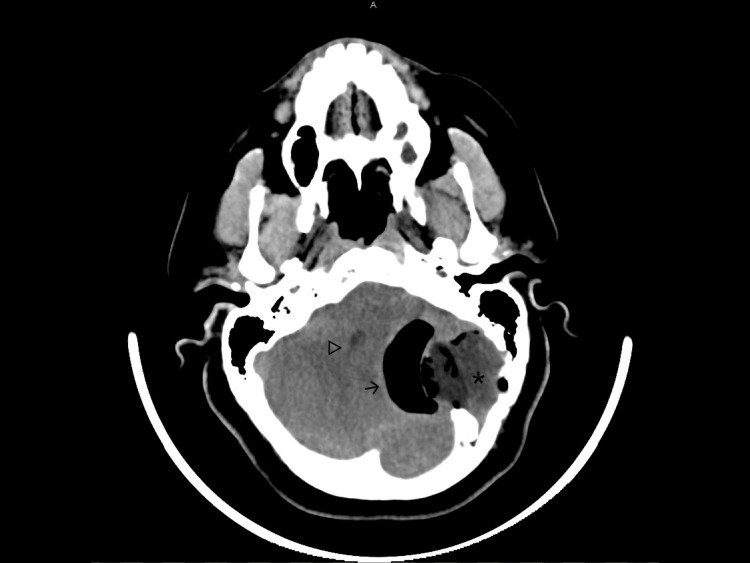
Preoperative CT scan showing a lesion (asterisk) originating from the intradiploic space and surrounded by intracranial air (arrow). It results in mass effect on the cerebellar hemisphere and compression of the fourth ventricle (triangle).
